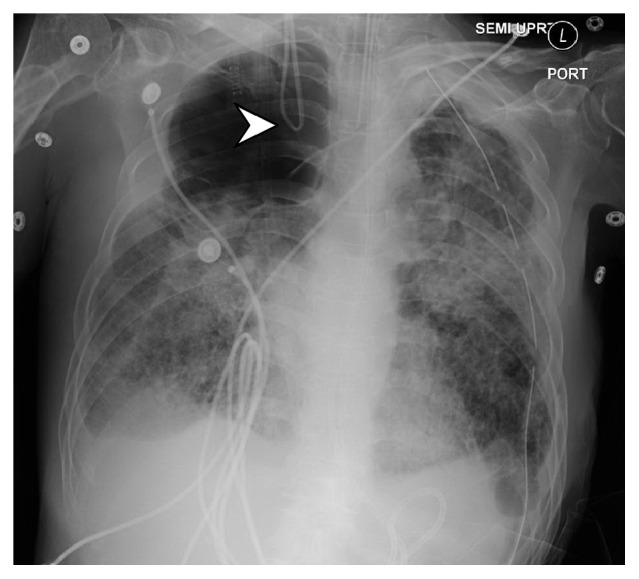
Portable chest x-ray with arrow depicting retrograde looping of pulmonary artery catheter.
